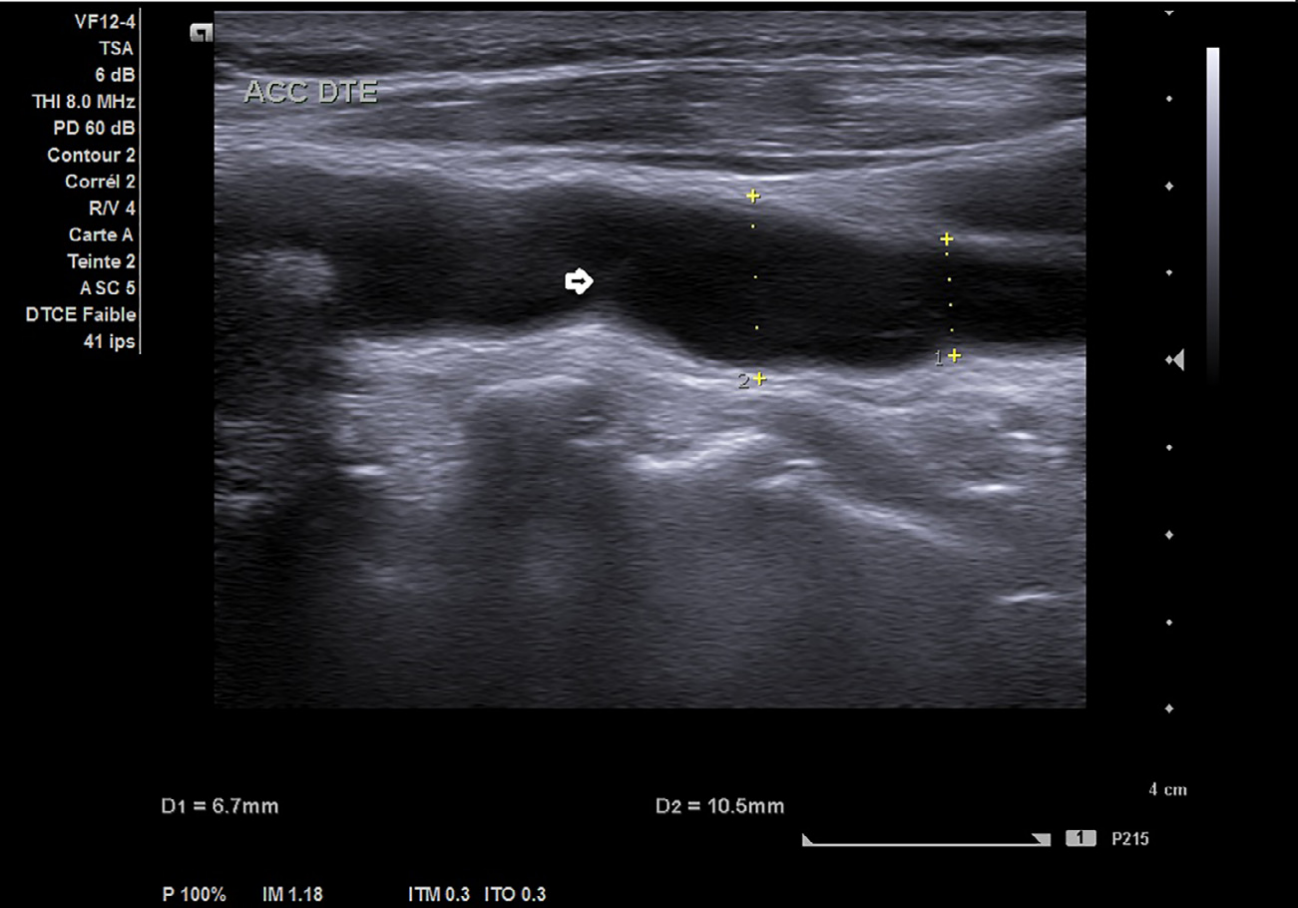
Color Doppler ultrasound scan showed an obviously localized dilation (arrow) with a thin diaphragm with a small central lumen on the distal part of CCA.
